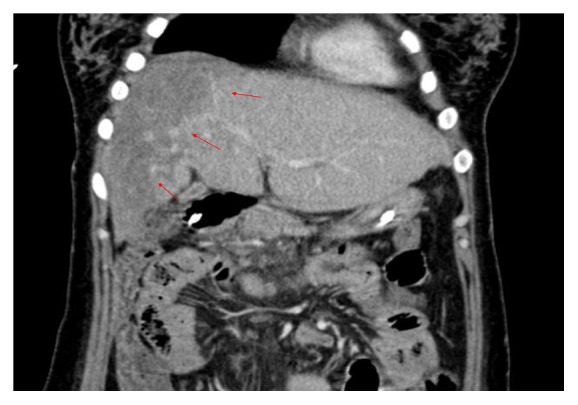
Pretransplantation CT scan showed a large area of infarction in the right lobe of the liver (arrows).
